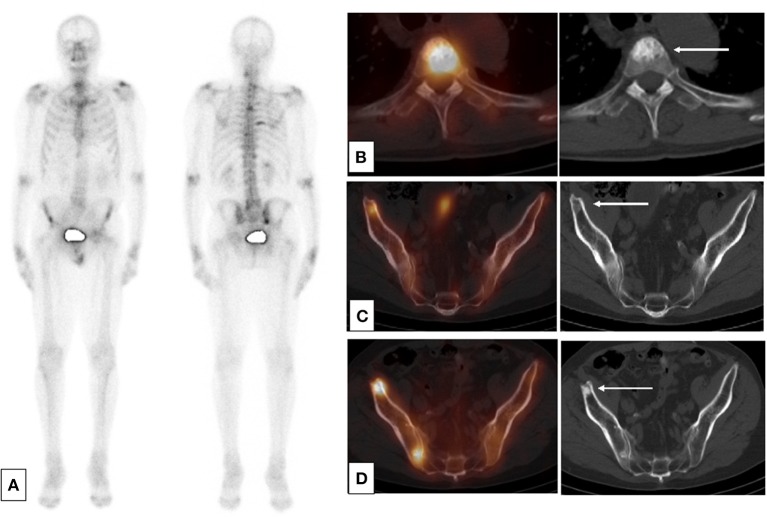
SPECT-CT: condensation of bone lesions under treatment. Occurrence of a prostate adenocarcinoma, Gleason 6 (3 + 3) on a biopsy, unoperated, treated by hormone therapy and HIFU therapy in a patient. Progressive re-elevation of the PSA 2 years after the cessation of hormone therapy. (A) Baseline planar bone scan (B,C) Baseline SPECT-CT and CT (axial slices) of lesions of T4 (B) and right ilium (C). (D) SPECT-CT and CT after 1 year of treatment by leuprorelin acetate showing an osteosclerotic reaction in the right ilium.
